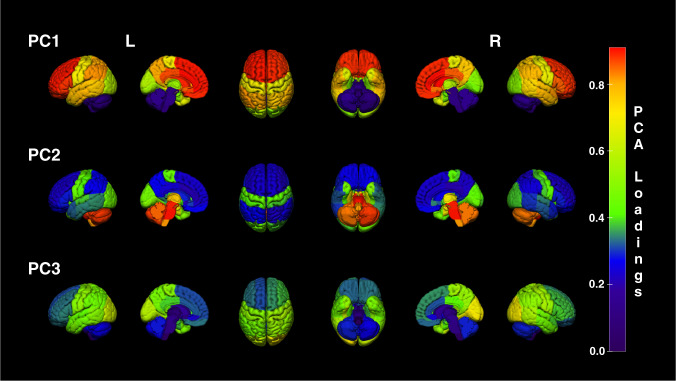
Representation of regional loadings from the first three PCs. Each Hammers atlas region was scaled to its loading score from the PCA. The 3D rendering clearly demonstrates the highest loadings for PC1 were obtained in frontal neocortex, followed by striatum (see Supplementary Fig. 4). Highest loadings for PC2 were found in the brainstem and cerebellum, which are often reference regions for SUVR calculations. Highest loadings for PC3 were found in the occipital cortex. The same data are tabulated in Supplementary Table 2. Colour bar represents PC loadings from highest (red) to lowest (violet). PC principal component, L left, R right.
